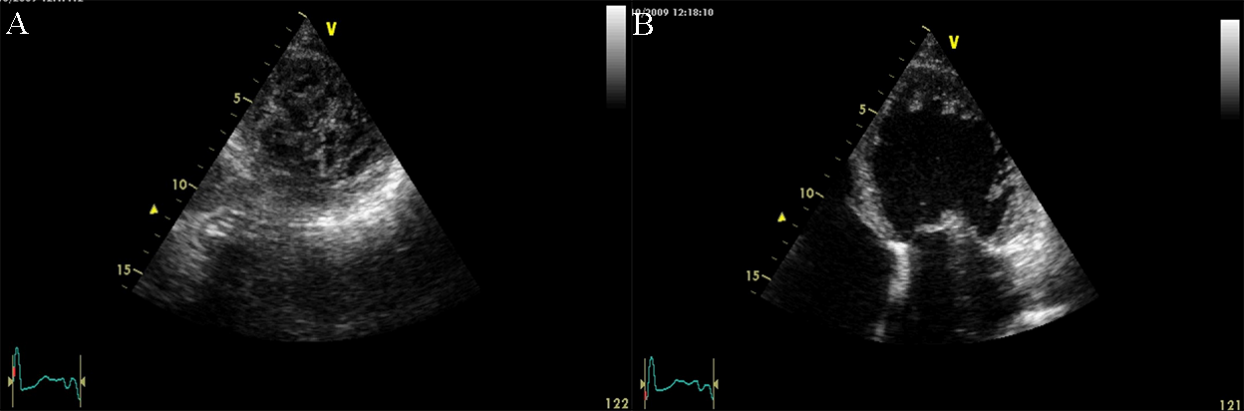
Echocardiographic non-compaction cardiomyopathy. (A) 2D short
